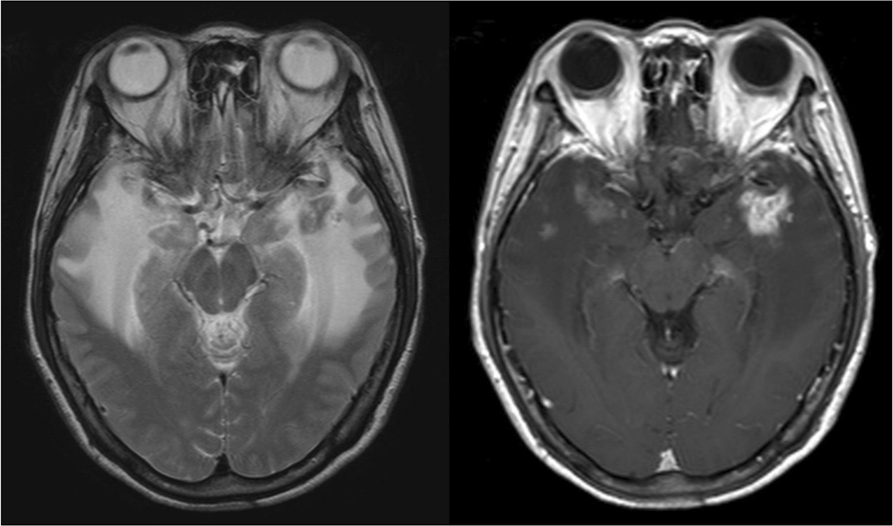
Cerebral radionecrosis shown by magnetic resonance imaging. T2-weighted image showing finger-like edema in both temporal lobes. Gadolinium-enhanced T1-weighted image of the same patient showing heterogeneous contrast enhancement.
